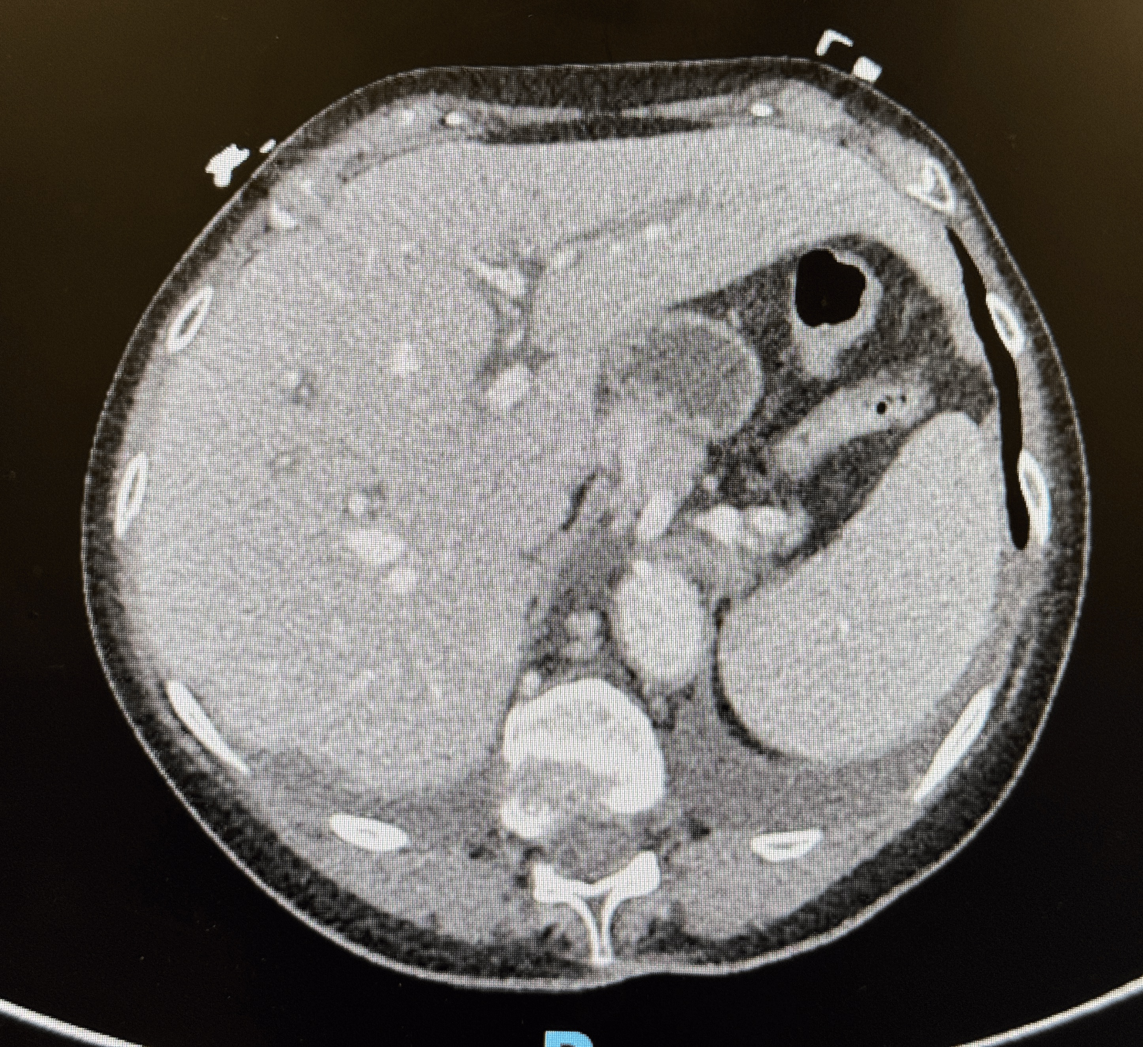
Abdominal CT showing hepatosplenomegaly.
